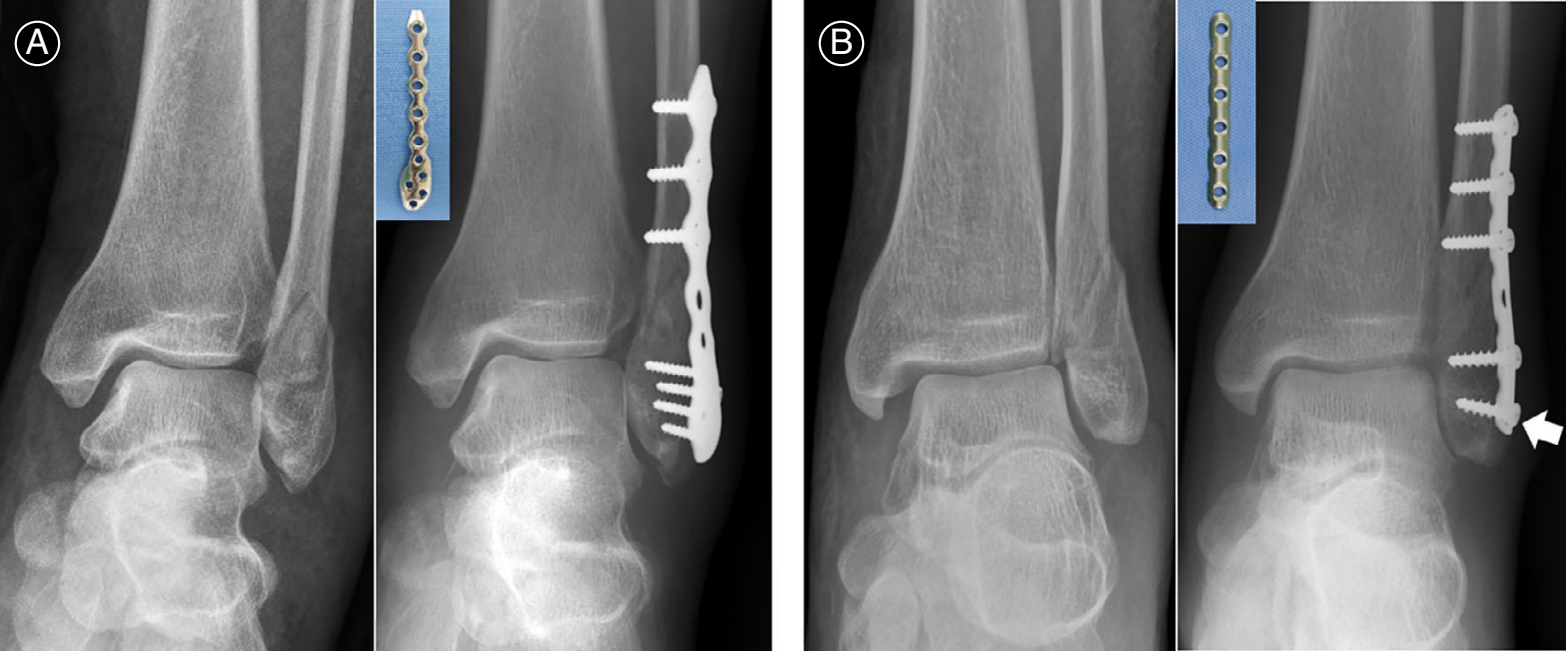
Radiographs of Danis‐Weber type B distal fibular fractures treated by plating osteosynthesis: (A) A 45‐year‐old female patient was treated with locking plate (LP) fixation. Three proximal 3.5‐mm bicortical non‐locking screws and four distal 2.7‐mm unicortical locking screws were used; (B) A 56‐year‐old male patient was treated with one‐third tubular plate (TP) fixation. Three 3.5‐mm bicortical non‐locking screws were used for proximal holes, while two 4.0‐mm unicortical cancellous screws were used for distal holes. Two technical tips are recommended to provide a good bone purchase of the distal fragment during TP fixation. First, the plate should be pre‐bent anatomically along the lateral aspect of distal fibula. Second, the most distal unicortical cancellous screw fixation should be slightly angled to prevent pulling out (white arrow).
